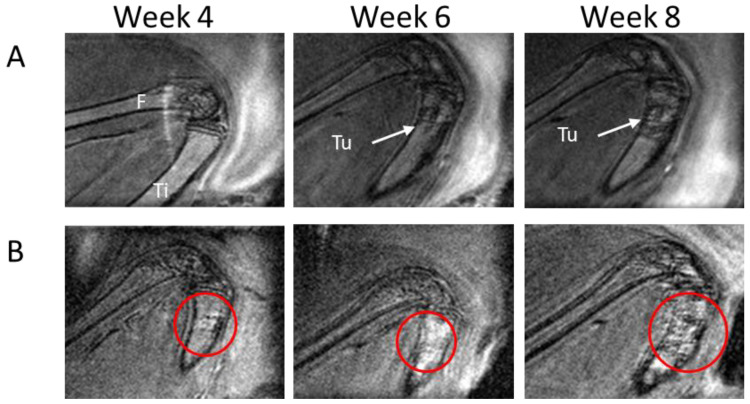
Tumor growth monitored with MRI. Representative images of tumor growth at the 4-, 6-, and 8-week MRI time points of (A) small tumors; (B) large tumors. F = femur, Ti = tibia, Tu = tumor. In B, red circles indicate tumor location.
